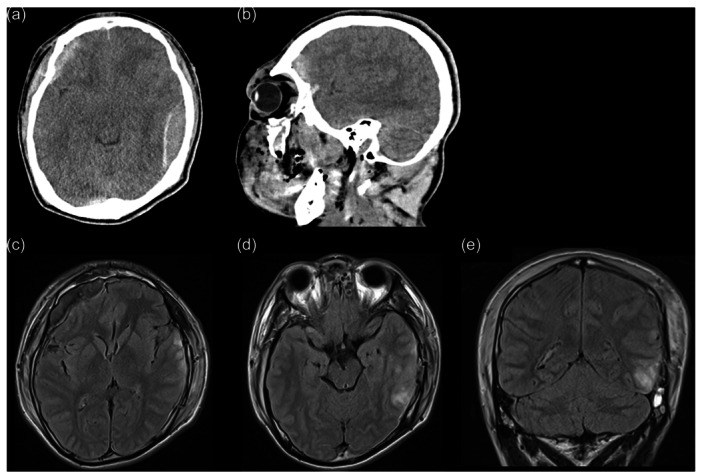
(a,b) Brain computed tomography immediately after head trauma reveals cerebral contusions of the right frontal and left temporal lobes, right frontal subdural hematoma, and left temporal epidural hematoma. (c–e) Fluid‐attenuated inversion recovery sequence brain magnetic resonance imaging 6 days after head trauma shows cerebral contusion of the right frontal and left temporal lobes and right frontal subdural hematoma.
